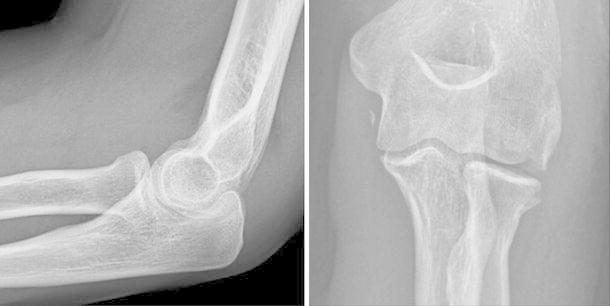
Anteroposterior and lateral radiographs of the injured elbow showing an avulsion fracture of the lateral epicondyle of the humerus
